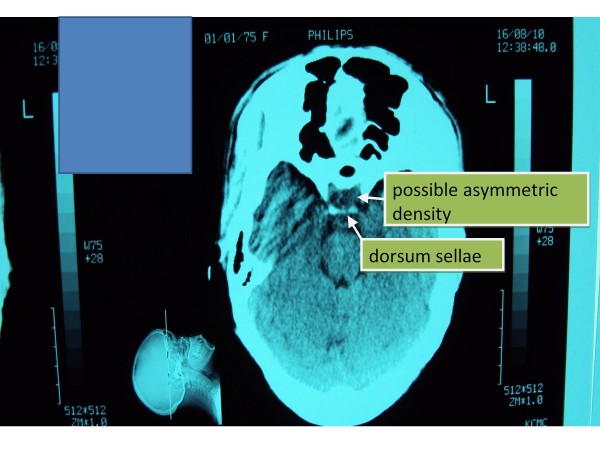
Non-contrast axial head CT scan*(at the level of sella). Legend: Possible asymmetric density within the pituitary gland on the left (but no ‘empty sella’ sign); dorsum sellae poorly visualized.
